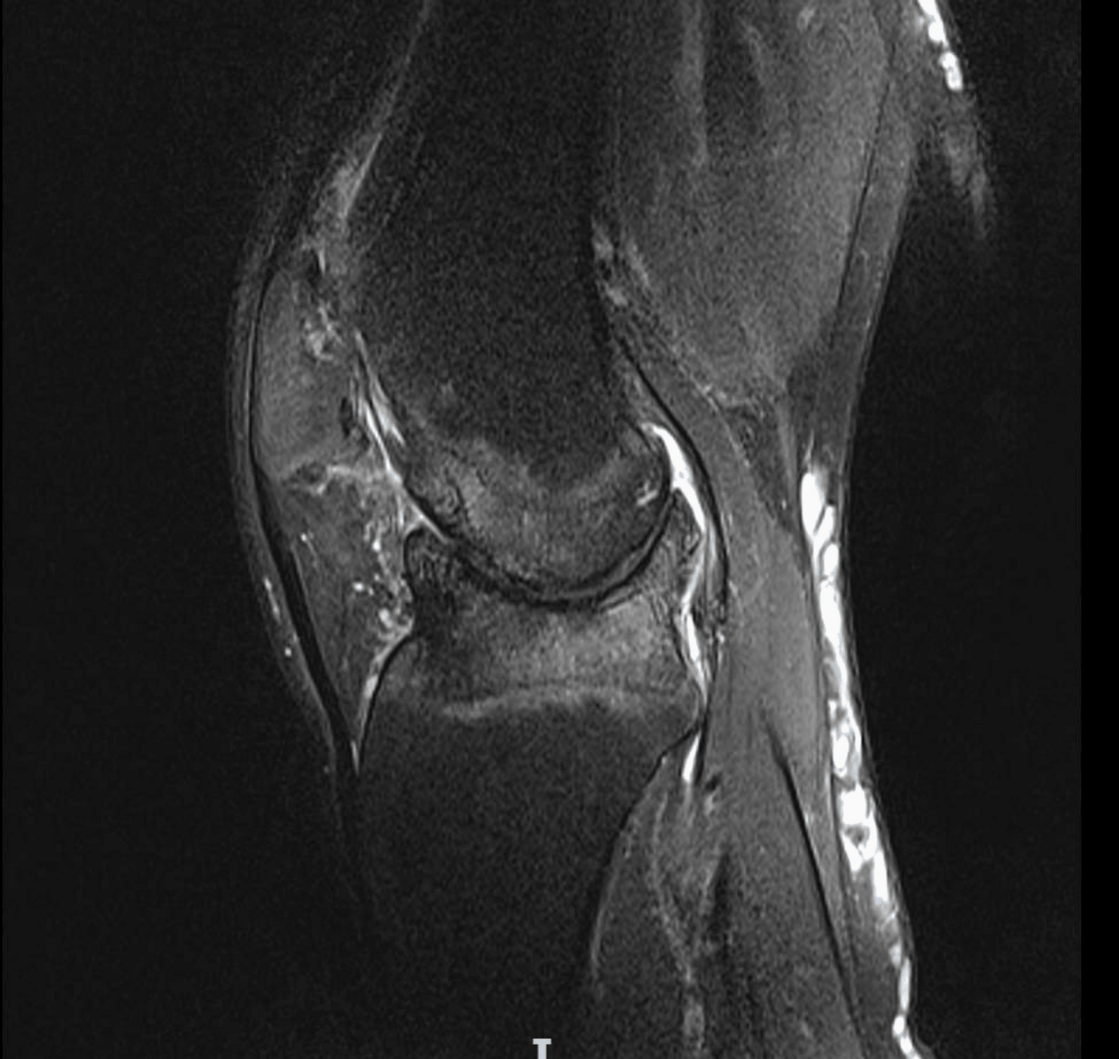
Preoperative sagittal MRI of the left knee.
